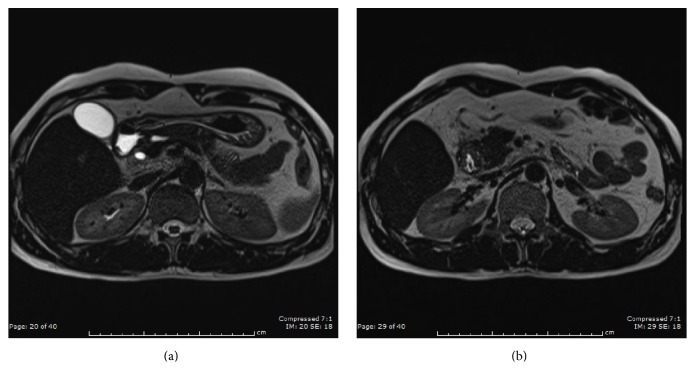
A follow-up MRI after 3 months of conservative treatment shows a nearly complete resolution of the duodenal hematoma.
